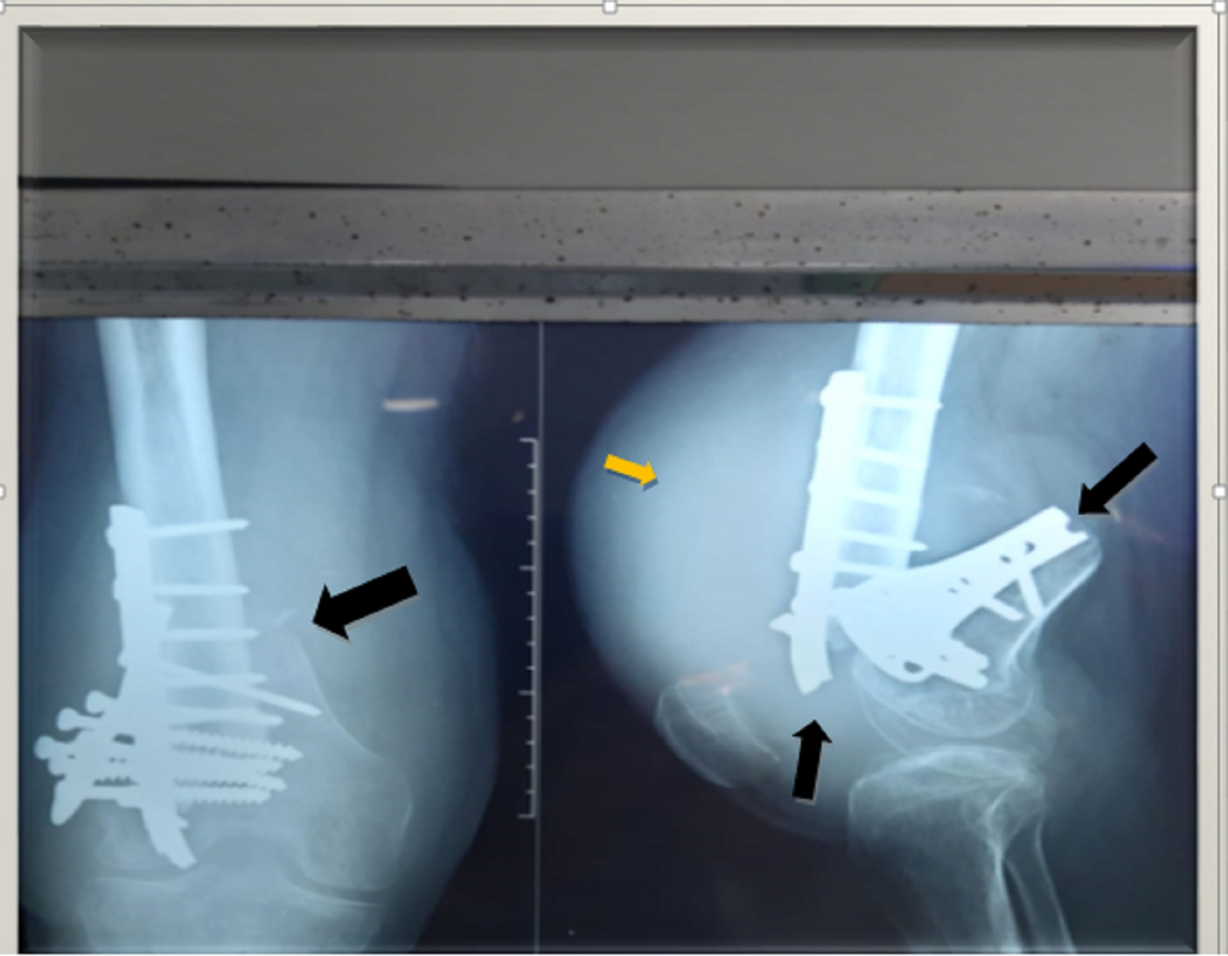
X-ray on the right shows the right distal femur in AP view, revealing implant breakage with a fracture of the femur (black arrow). The image on the left shows a lateral view of the right-sided distal femur, clearly revealing an implant breakage (black arrows) along with soft tissue swelling (yellow arrow). AP, anteroposterior
